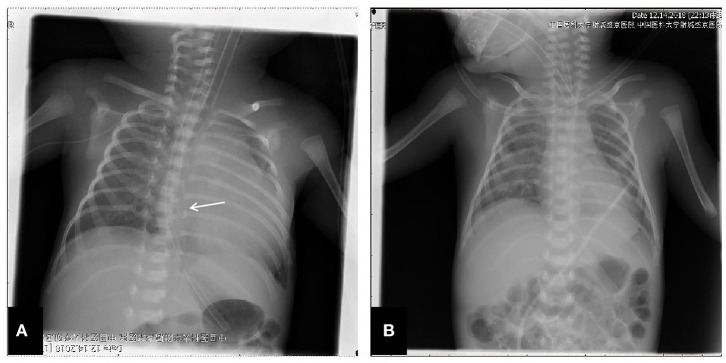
Chest X-ray presentation. (A) Chest X-ray performed at the 8th day after birth, which showed the distorted catheter as well as enlarged heart shadow; (B) Chest X-ray performed after the removal of PICC and the performance of pericardiocentesis, and the heart shadows were greatly reduced.
